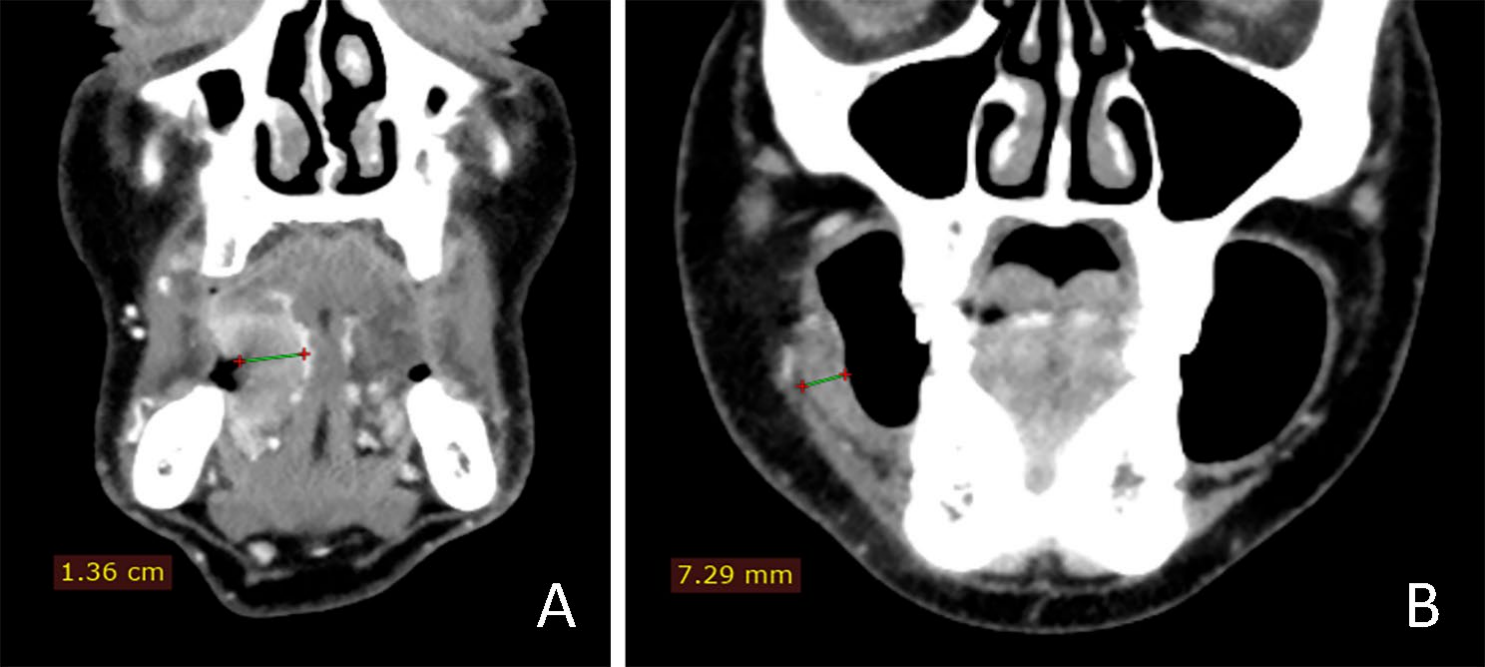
(A) Depth of tumor invasion (DOI) measured on the coronal reformatted contrast-enhanced CT image of the tongue OSCC. The tumor presented as a postcontrast hyperdense soft tissue mass. (B) DOI measured in the OSCC of the buccal mucosa. The tumor presented as an irregular thickening of the buccal and inferior fornix mucosa
